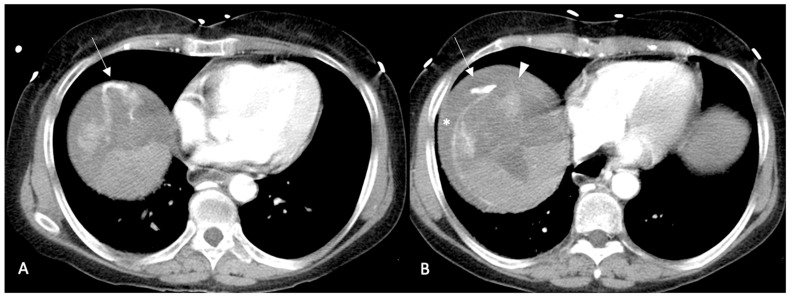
Arterial phase enhanced CT scan. HCC of the VII–VIII segment with intra- and extracapsular hemorrhage and active contrast extravasation in the context of peritoneal effusion ((A,B) arrows). (B) Interruption of the Glissonian capsule (arrowhead) with concomitant hemoperitoneum (asterisk) is clearly visible.
